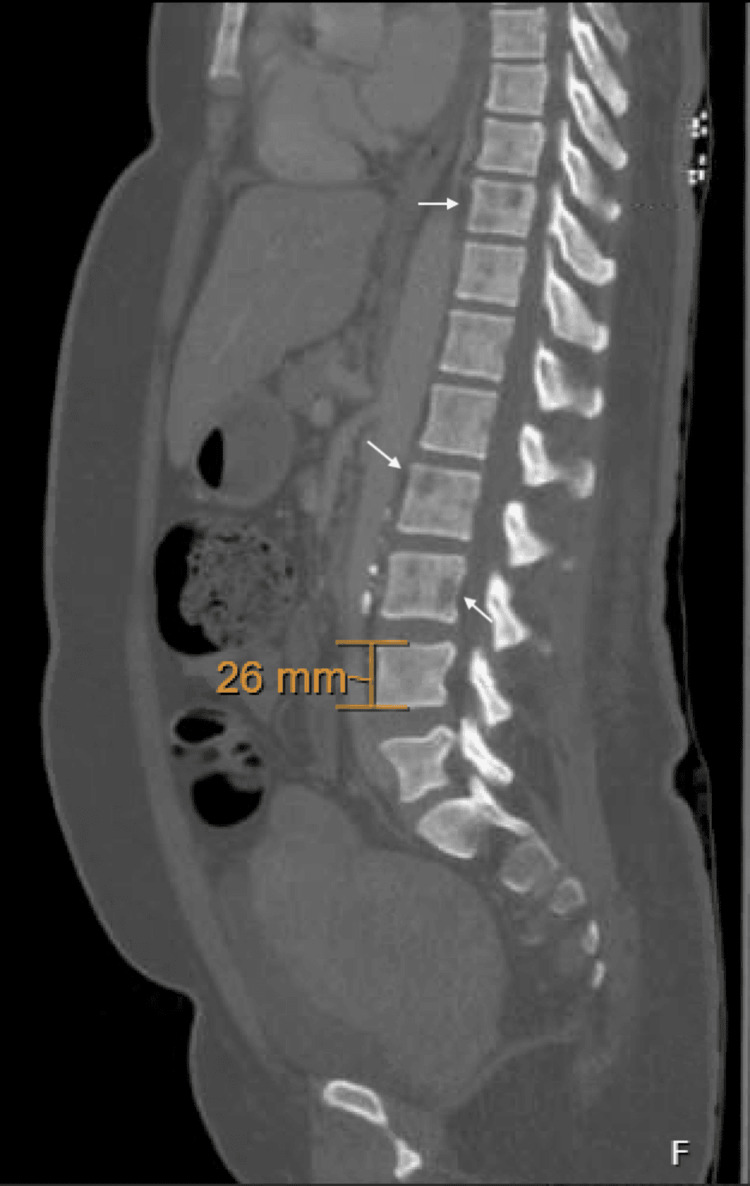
CT scan of the abdomen and pelvis (sagittal view) demonstrating innumerable lytic lesions on multiple levels of vertebral bodies (white arrows)
